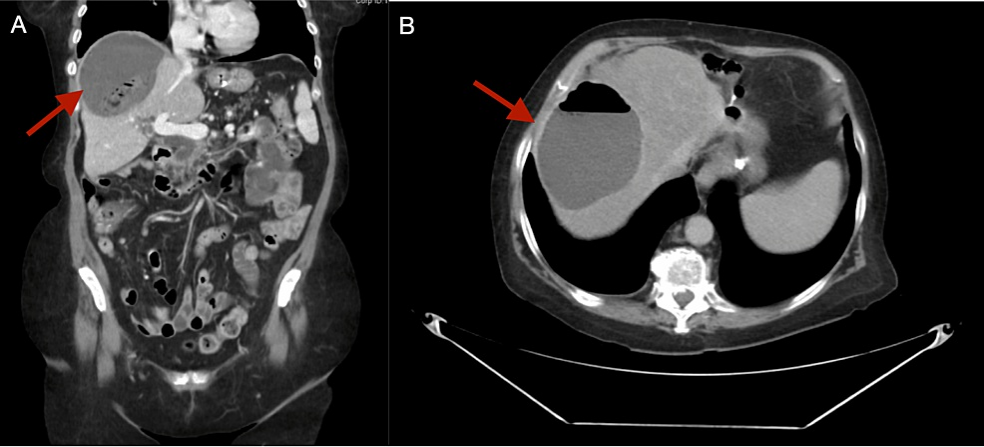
CT of the abdomen and pelvis with the portal venous phase of contrast enhancement performed on admission (one week after the previous CT scan) showing a 10.3 × 8.4 × 9.1 cm air/fluid collection of the liver. (A) Coronal view. (B) Axial view. CT: computed tomography
